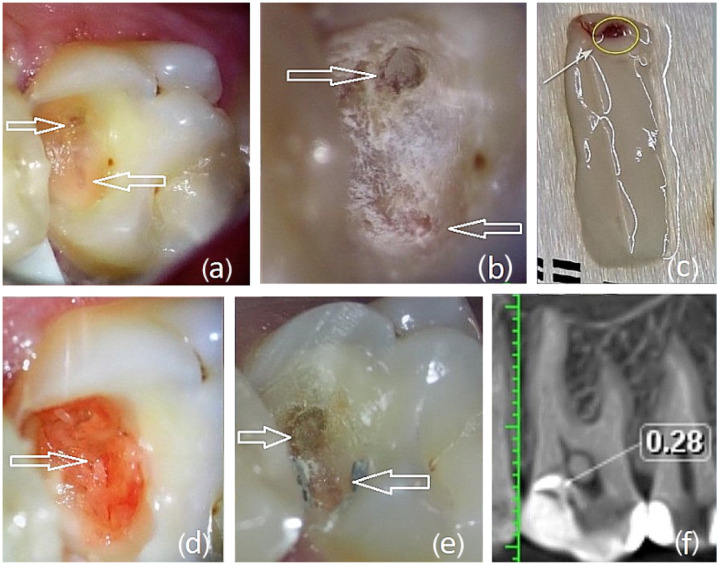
Patient,16 years old, with reversible pulpitis treated by direct pulp capping. (a) After removing the infected dentin, the white arrows indicate multiple pulp exposures. (b) Following ozonation, the white arrows mark the pulp exposure in dentin; the palatal pulp horn exposure lies below the level of the surrounding dentin. (c) The area of the H-PRF membrane used to cover the pulp communication is indicated by a yellow circle. (d) The exposure was covered with a H-PRF membrane—the white arrow on the axial wall of the cavity. (e) After removing the temporary restoration (day 46), the sites previously marked by white arrows were filled with reparative dentin. (f) CBCT (sagittal slice, cropped) of tooth 16 (after day 46) showing no communication with the dental pulp. The reparative dentin had a thickness of 0.28 mm.
